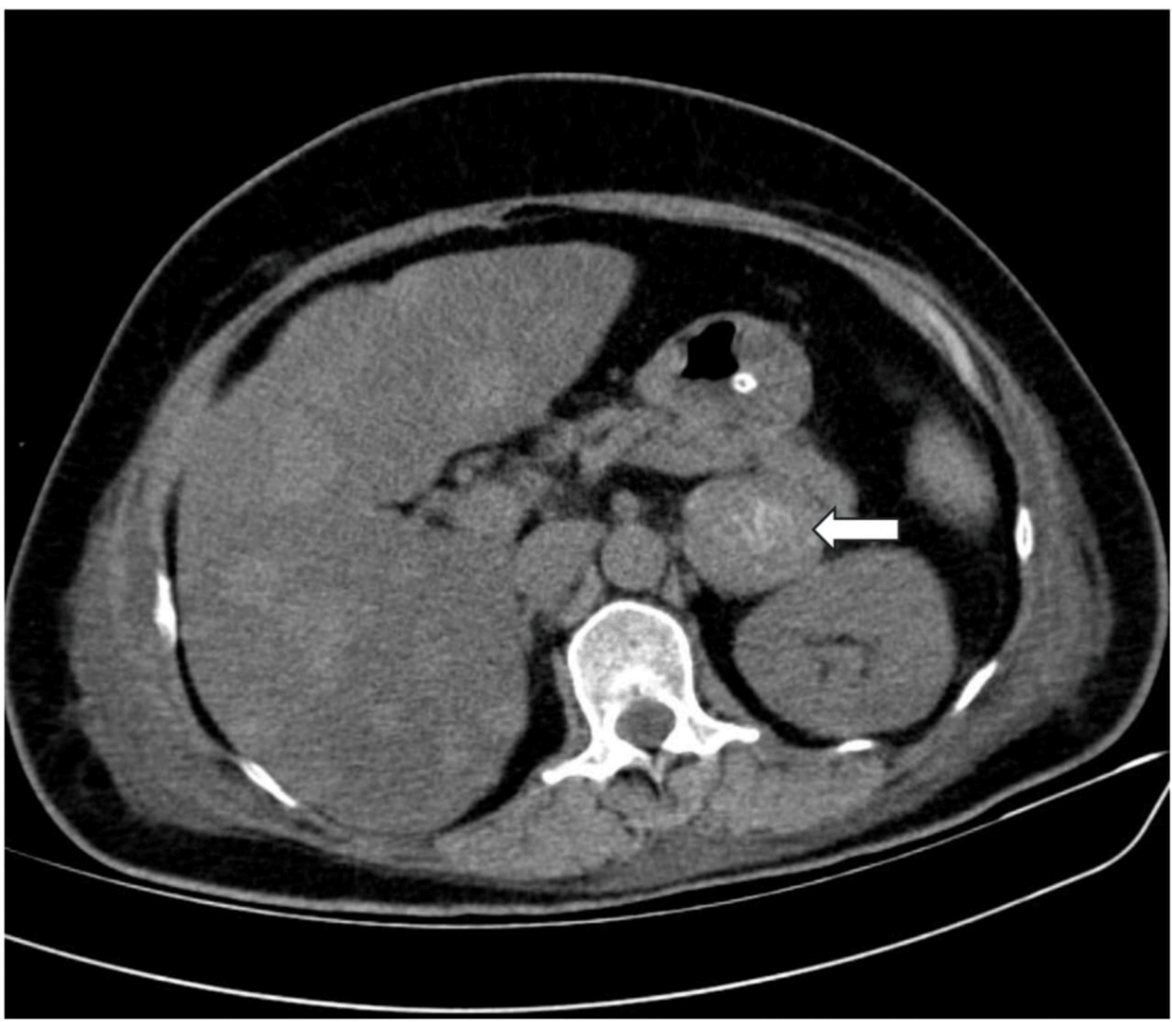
CT abdomen axial section showing a left suprarenal mass (white arrow)
